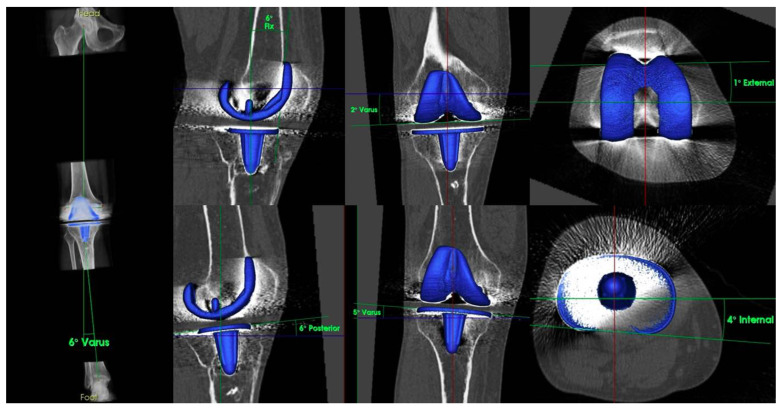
TKA component position in 3D reconstructed CT scans.
